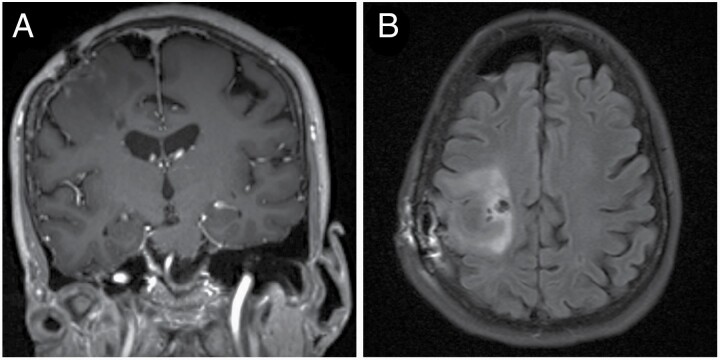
MRI of recurrent IDH^mut^ glioma. (A) Coronal T1 with gadolinium showed an ill-defined lesion in the right frontal perirolandic area with equivocal contrast enhancement. (B) FLAIR in axial plane.
